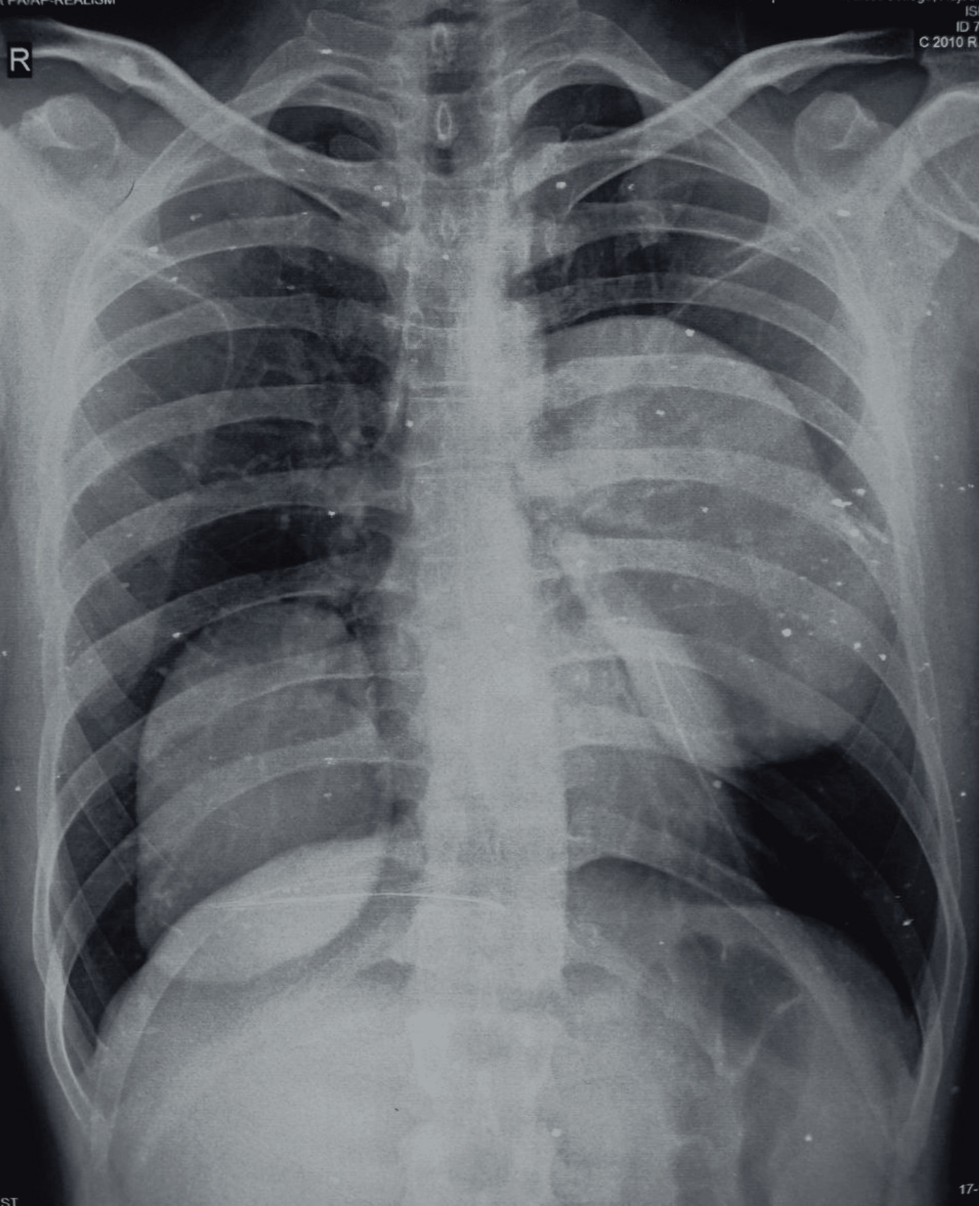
Chest X-ray showing hypodense lesions in both lobes
